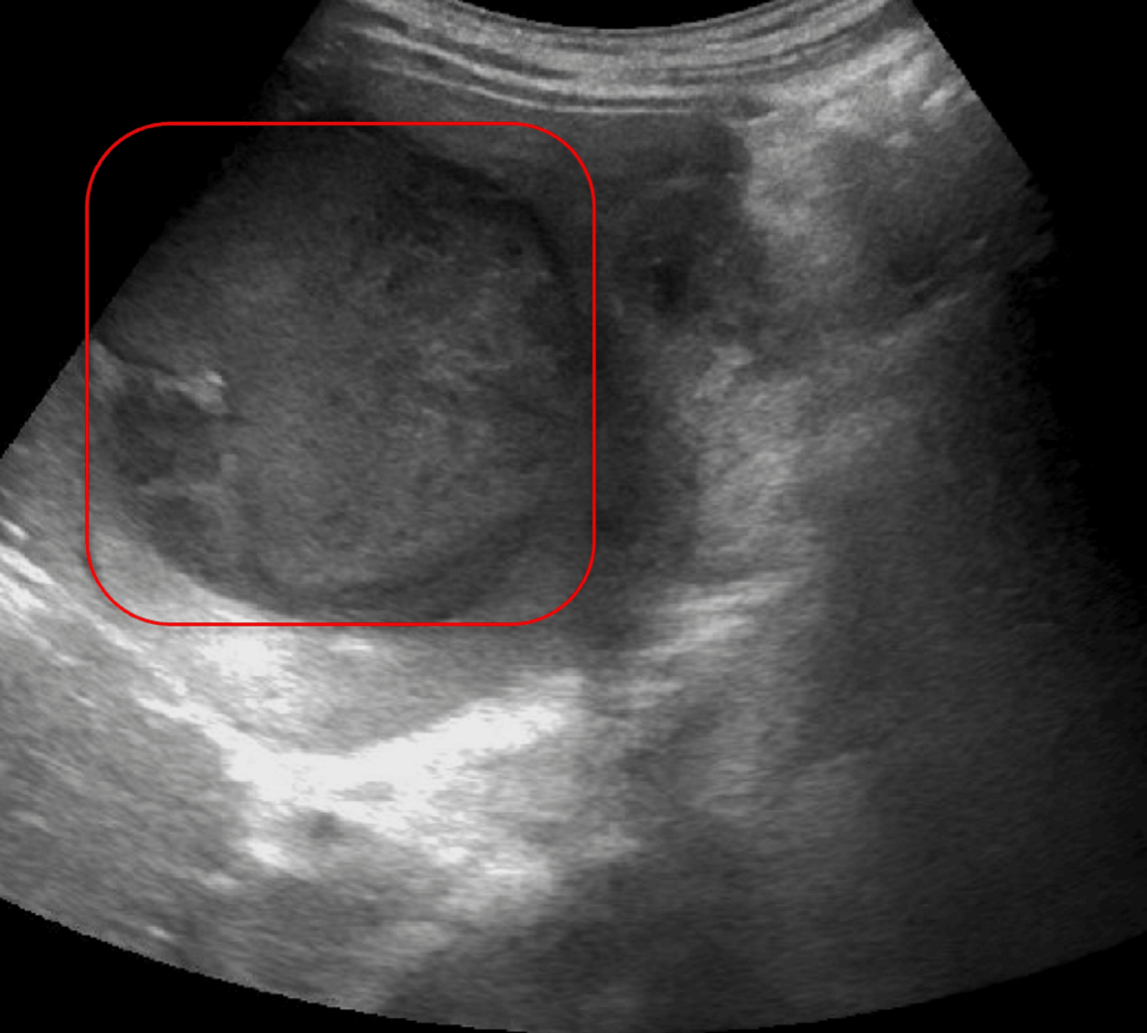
RUQ ultrasound with a complex fluid collection in the right hepatic lobe (red square). RUQ: right upper quadrant
